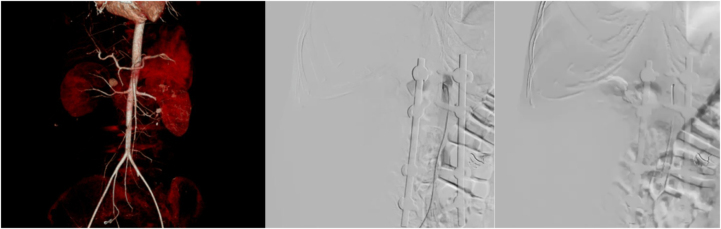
CT Angiography demonstrated a right lumbar artery pseudoaneurysm and embolization of the pseudoaneurysm was performed.
